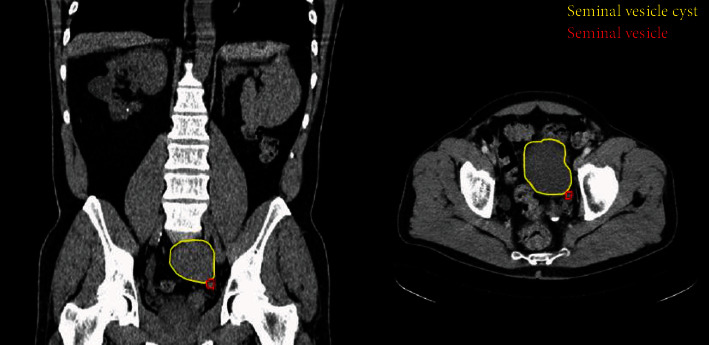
CT, axial, and coronal sections from scan in 2004, of a more inferior aspect of the cyst (outlined in yellow), identifying its relationship to the seminal vesicle (outlined in red).
